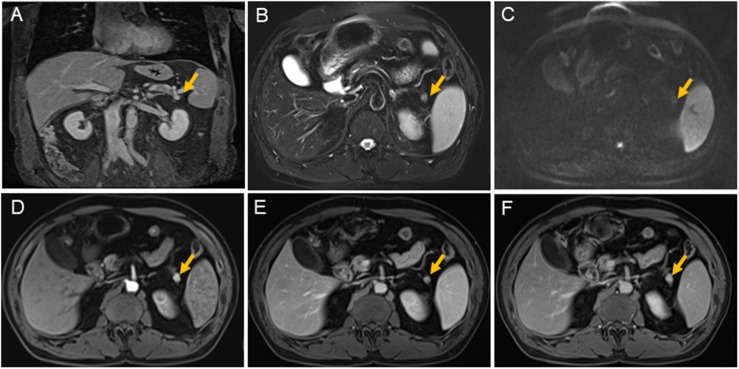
Magnetic resonance imaging scans of the pancreas. There is a nodule about 1.1 cm × 1.2 cm (yellow arrow) with clear margin in pancreatic tail. The nodule is shown isointensity on T1 weighted-image (A); on T2 weighted-image, it is demonstrated hyperintensity (B); it is shown slight restricted on diffusion weighted image (C); it is shown contrast-enhancement on early arterial phase (D); also on balance phase (E); and continuing contrast-enhancement on venous phase (F). The main pancreatic duct is not expanded. The size and form of liver, gallbladder, and spleen is not unusual, the signal is normal.
